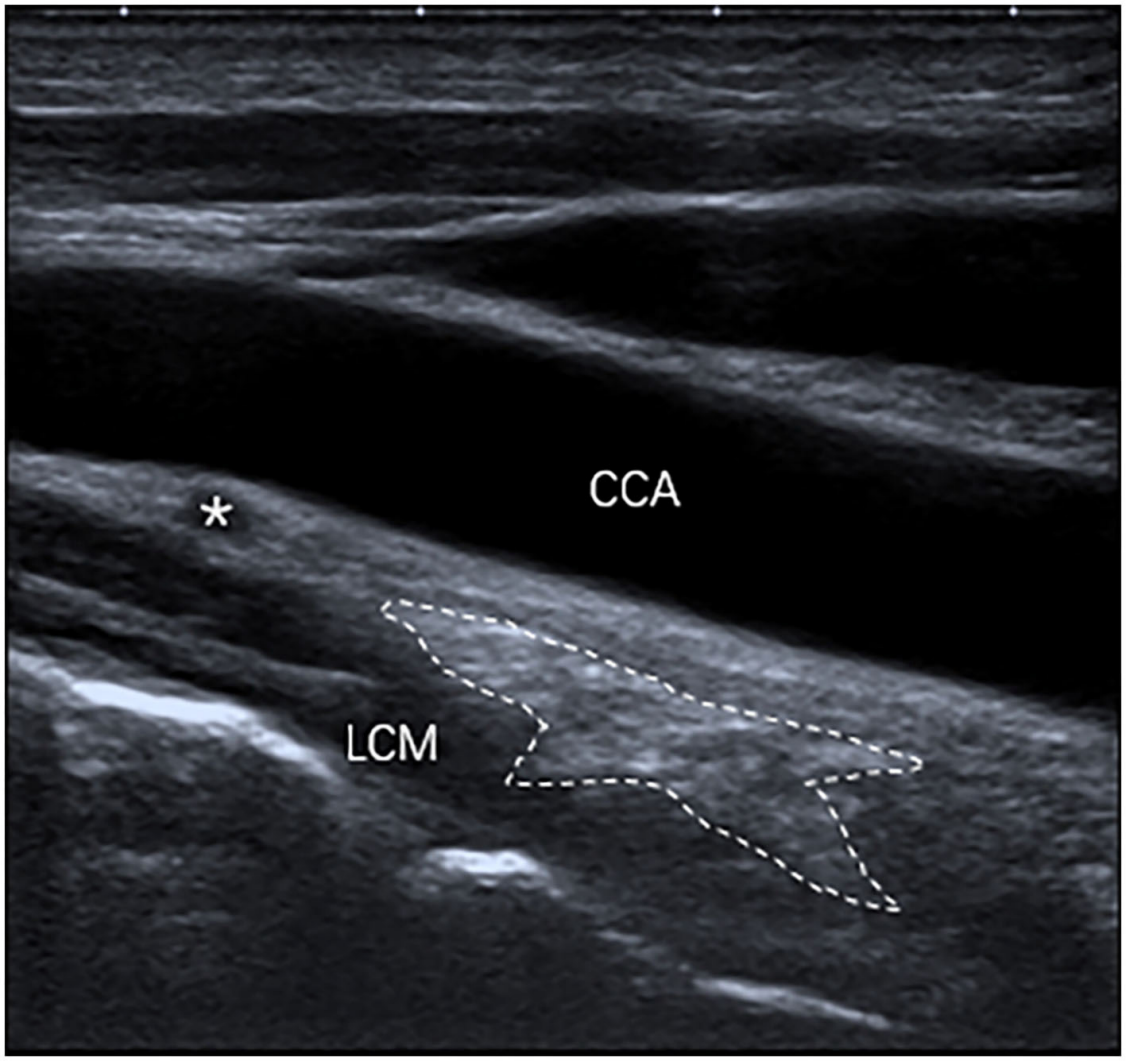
The SG, with a star shape (outlined) and hyperechogenicity, was located between the common carotid artery (CCA) and the longus colli muscle (LCM) and was under the inferior thyroid artery (*) on the longitudinal ultrasound image.
